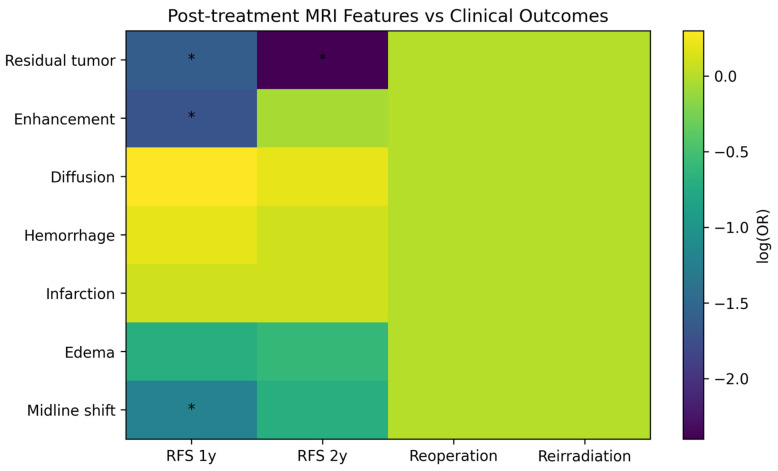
Integrated overview of post-treatment MRI predictors across clinical outcomes. Asterisks (*) indicate cells reaching the conventional significance threshold (*p* < 0.05) in the corresponding regression model.
